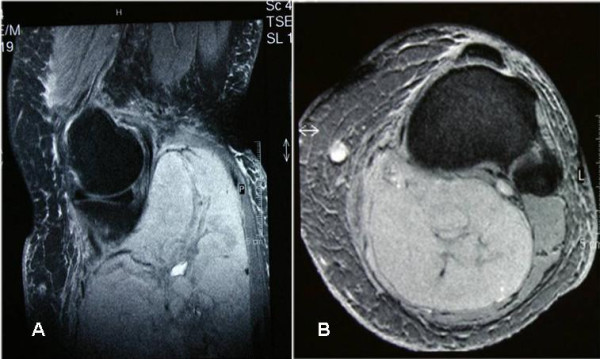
A & B – MR images (longitudinal & transverse sections) of the left leg (case 1) showing a massive pleomorphic sarcoma involving the whole posterior compartment.
